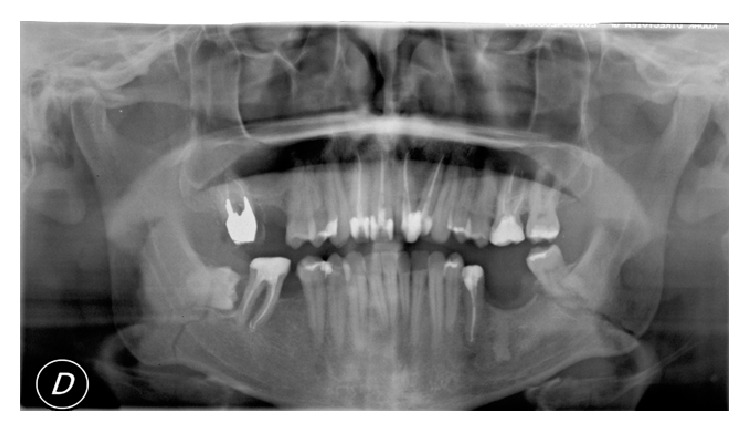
Preoperative panoramic radiograph showing M3 in the fracture lines.
